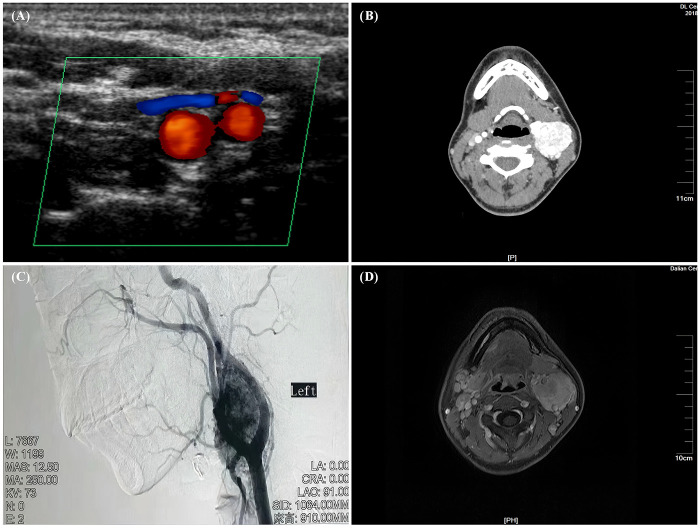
Preoperative multimodal imaging findings supporting the diagnosis and retrospective shamblin assessment of the left carotid body paraganglioma. (A) Preoperative color Doppler ultrasonography shows a hypervascular mass at the left carotid bifurcation. (B) Axial contrast-enhanced computed tomography angiography (CTA) demonstrates an intensely enhancing mass centered at the left carotid bifurcation with splaying of the internal and external carotid arteries. (C) Preoperative digital subtraction angiography (DSA) demonstrates marked tumor blush of a hypervascular lesion at the left carotid bifurcation. (D) Axial contrast-enhanced magnetic resonance imaging (MRI) demonstrates a heterogeneously enhancing carotid-space mass with internal flow-void foci. Taken together, the carotid bifurcation epicenter, splaying of the internal and external carotid arteries, hypervascular tumor blush, and close relationship to the carotid branches support carotid body origin and a retrospective assessment most consistent with Shamblin II.
